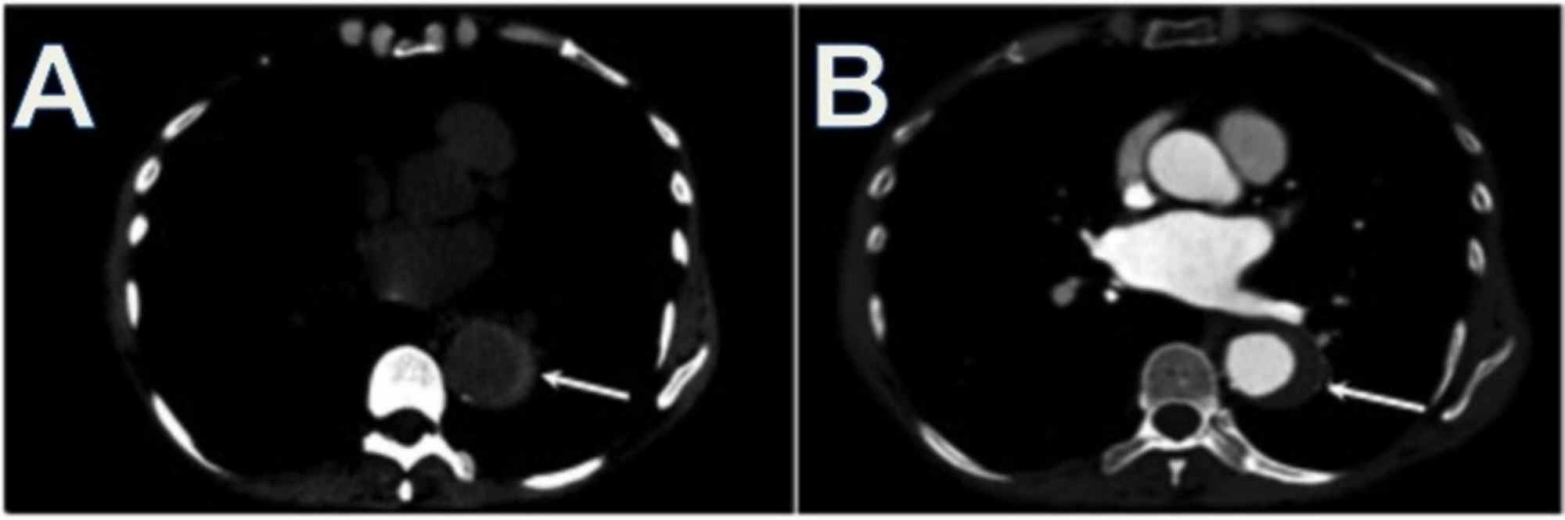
Intramural Hematoma A. Intramural hematomas is visualized as a crescent-shaped or ring like hyperdensity on non-contrast enhanced CT imaging.
